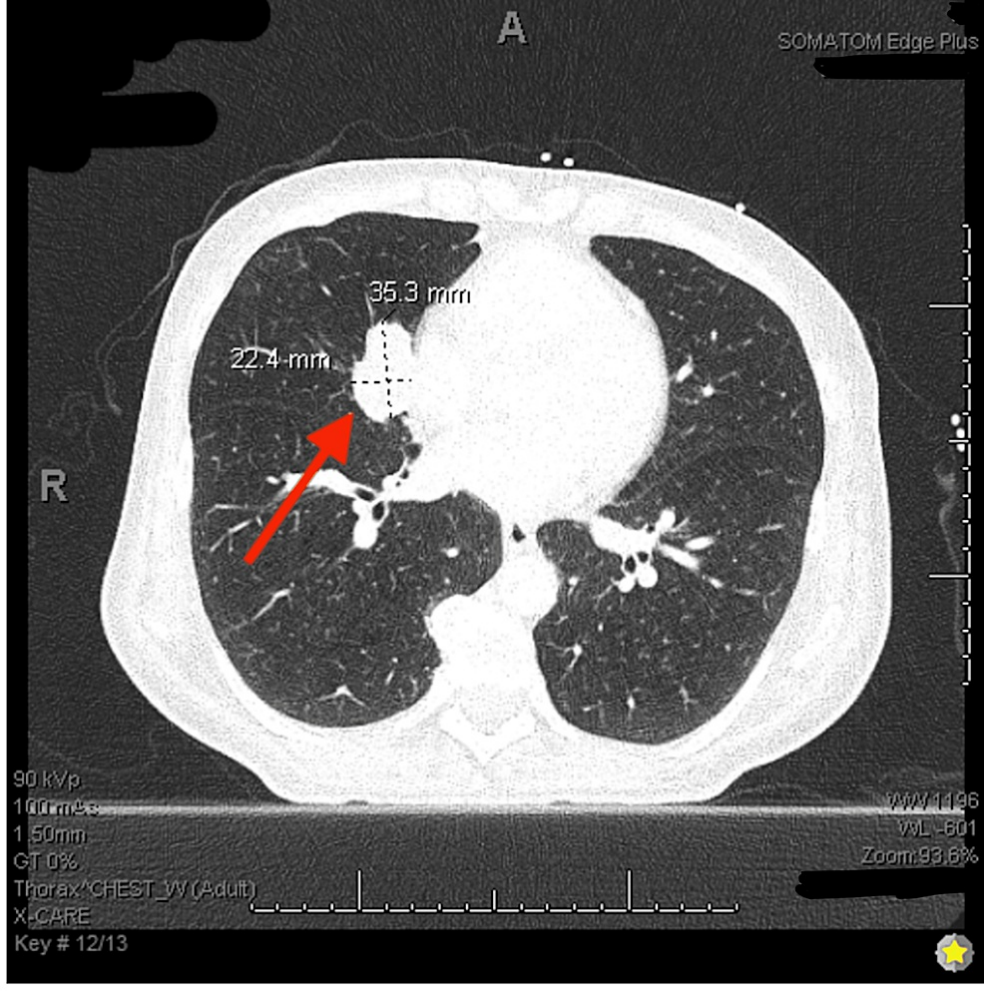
CT chest with iodine contrast showing right middle lobe lung mass of 2.2 x 3.5 cm.
